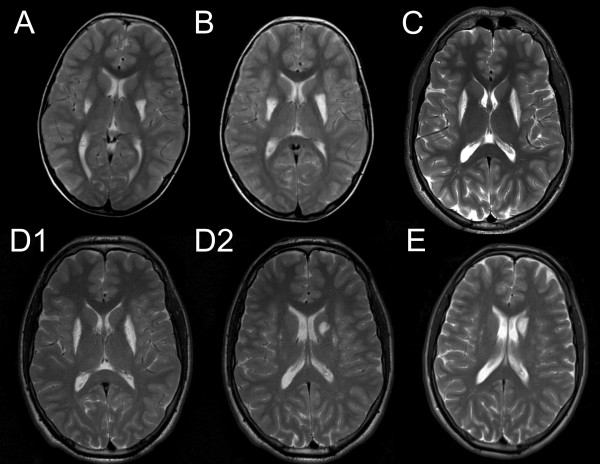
Axial T2-weighted MRI showing progression of the striatal lesions at the ages of nine (A), ten (B), fourteen (C), seventeen (D1&D2) and eighteen (E) years.
